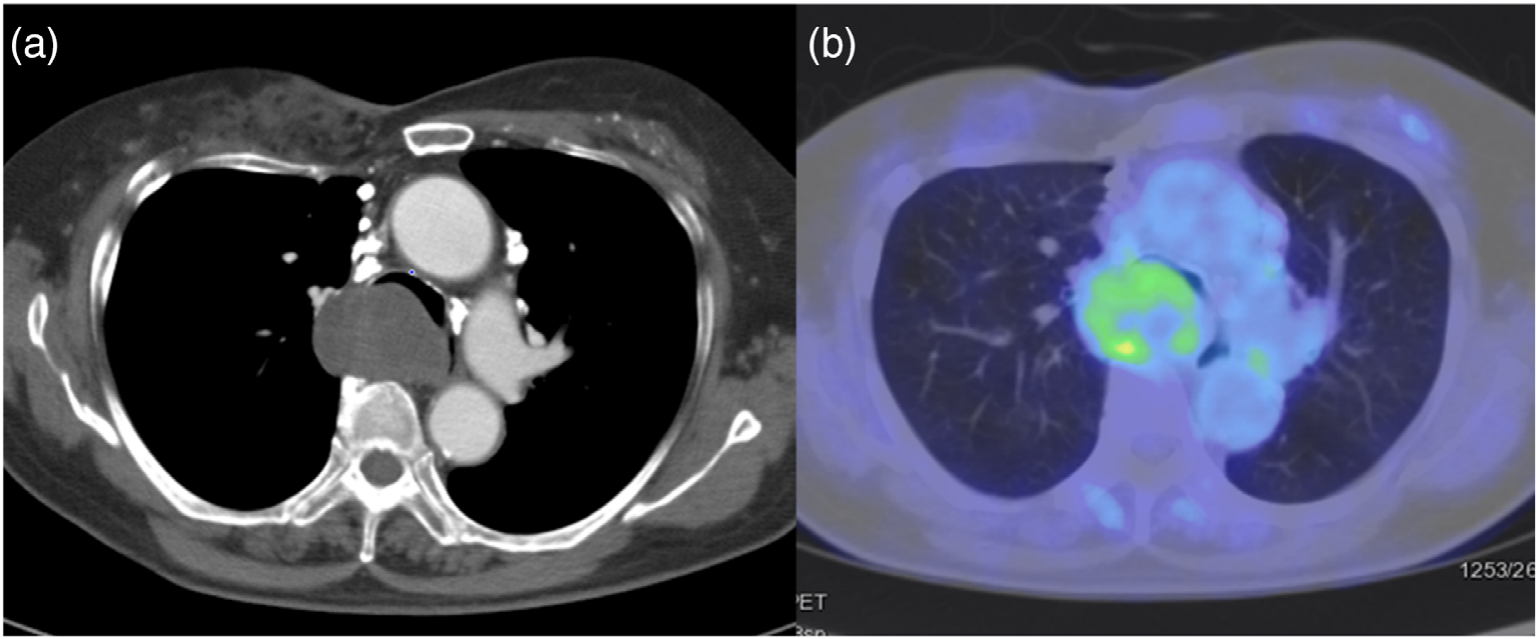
Chest computed tomography (CT) and positron emission tomography (PET)‐CT on the initial visit. (a) Chest CT showing a well‐circumscribed, homogeneous, 5‐cm sized middle mediastinal tumor (axial image). (b) PET‐CT showing a maximum standard uptake value of 3.4 on the mediastinal tumor (axial image).
